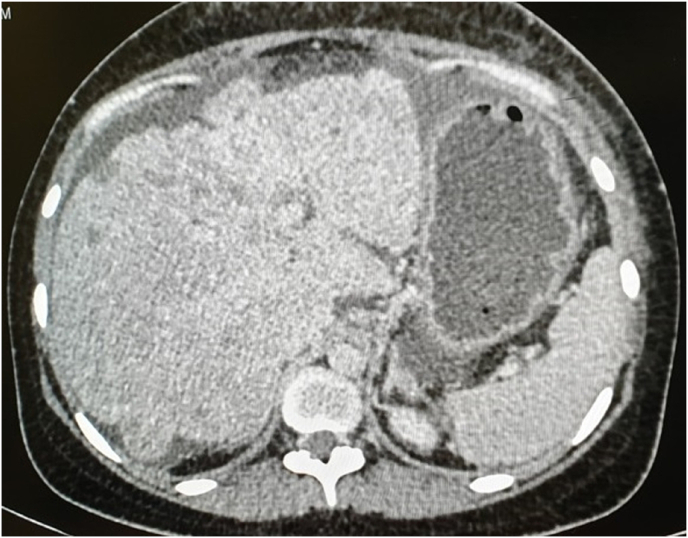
CT showing washout in delayed phase and ascites. Legend: Washout in delayed phase and ascites.
